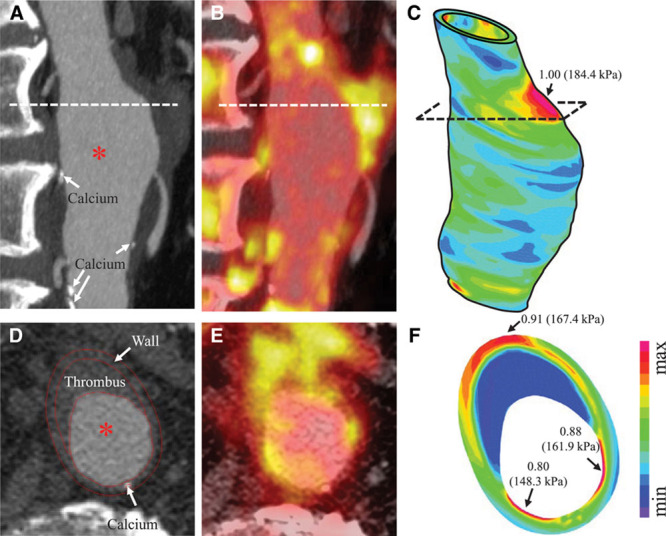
In vivo images and the calculated structural stress of an abdominal aortic aneurysm: (A) contrast-enhanced computed tomography (CT), sagittal view; (B) fluorine-18-labeled 2-deoxy-2-fluoro-d-glucose (^18^F-FDG) positron emission tomography (PET), sagittal view; (C) structural stress plotted on the 3-dimensional geometry (both normalized and absolute values); (D) contrast-enhanced CT, transverse view; (E) ^18^F-FDG PET, transverse view; and (F) structural stress plotted on the transverse plane (both normalized and absolute values).
